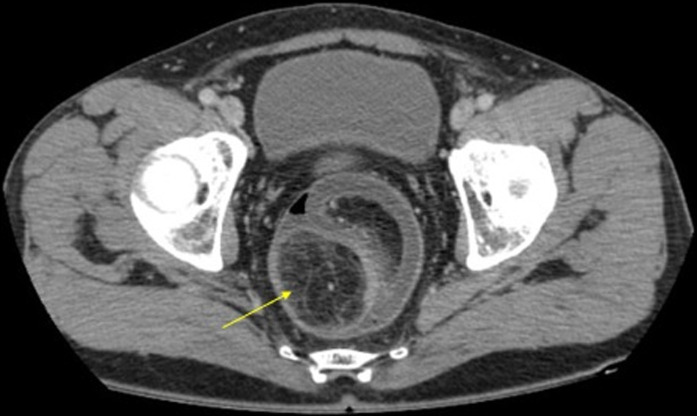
axial view of a CT scan of the abdomen showing a lipoma of 6cm of diameter filling the rectum and being the lead point to a colorectal intussusception (yellow arrow)
